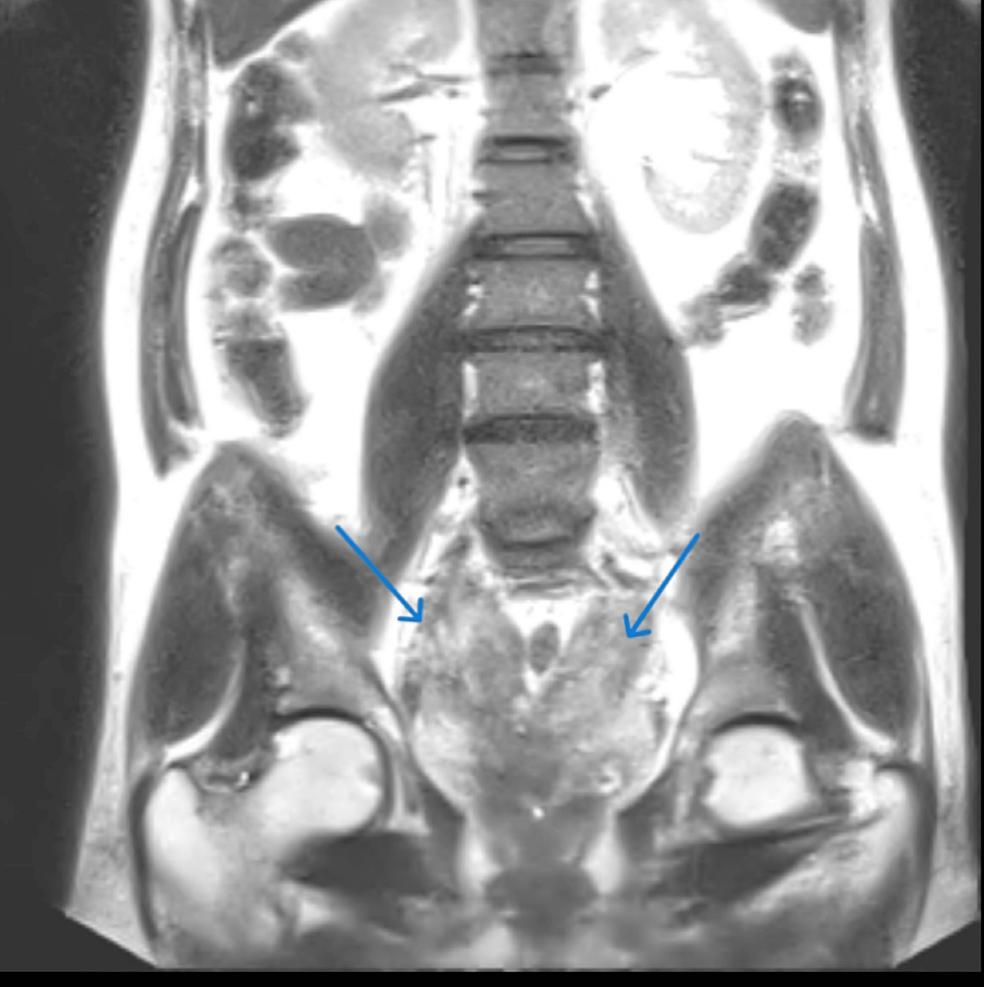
MRI of the pelvis showing a mass involving bilateral seminal vesicles and abutting the posterior wall of the urinary bladder.
